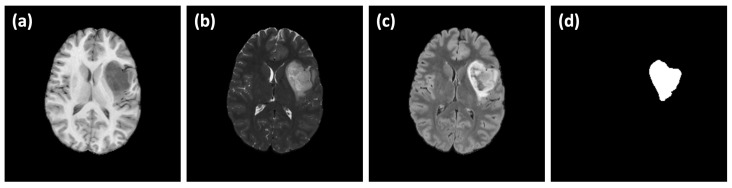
Sample of the UCSF-PDGM dataset. (a) T1w, (b) T2w and (c) FLAIR MRI scans with (d) astrocytoma segmentation label.
